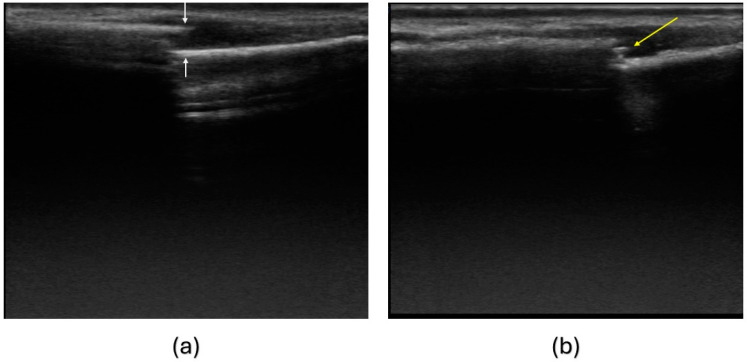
Ultrasound examination of a patient. (a) The ultrasound image on the 4th day after the injury showed no visible callus. The white arrows point to fracture ends. (b) Ultrasound image on the 8th post-injury day, with the yellow arrow pointing to the callus formation.
